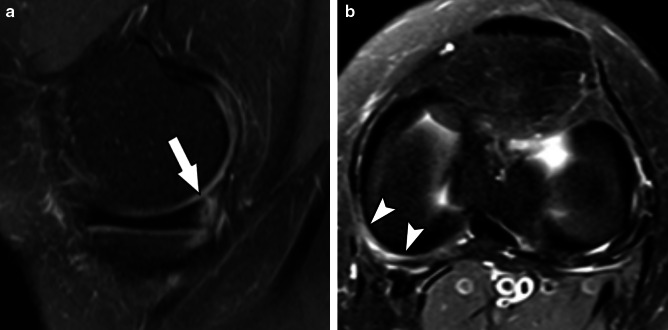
Type 1 meniscal ramp lesion MRI Case. Sagittal (a) and axial T2-weighted fat-suppressed images show meniscocapsular tear (arrow) with tibial bone bruise, and the extension of meniscocapsular junction edema (arrowheads)
